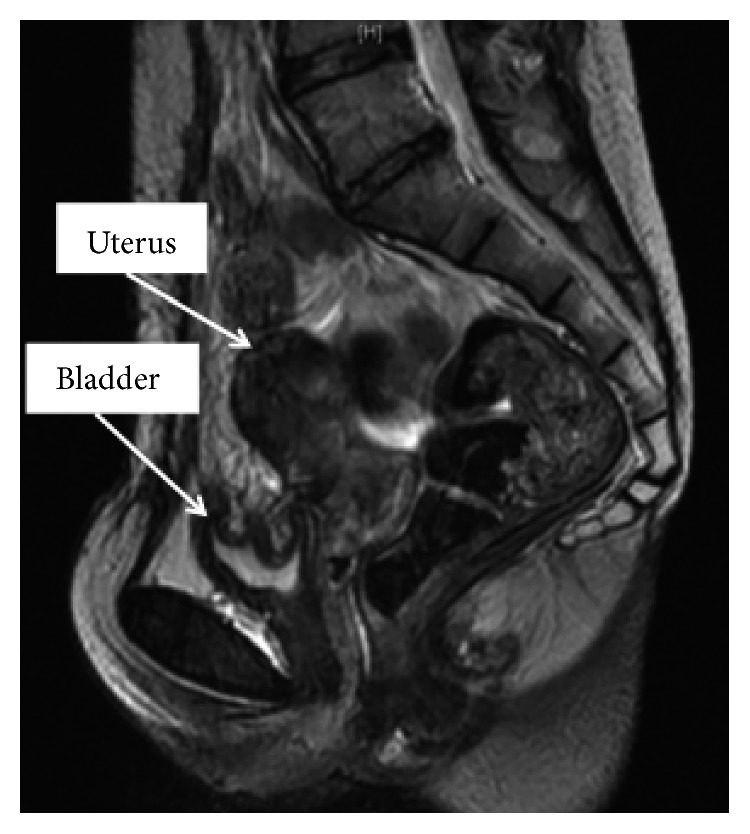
MRI image taken 4 months after drainage operation. Abscess or cyst could not be detected.
